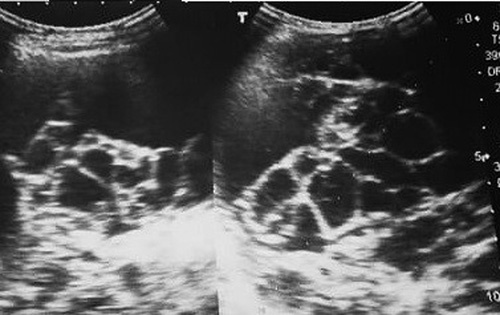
Abdominal ultrasound revealing a well-marginated multicystic mass.
